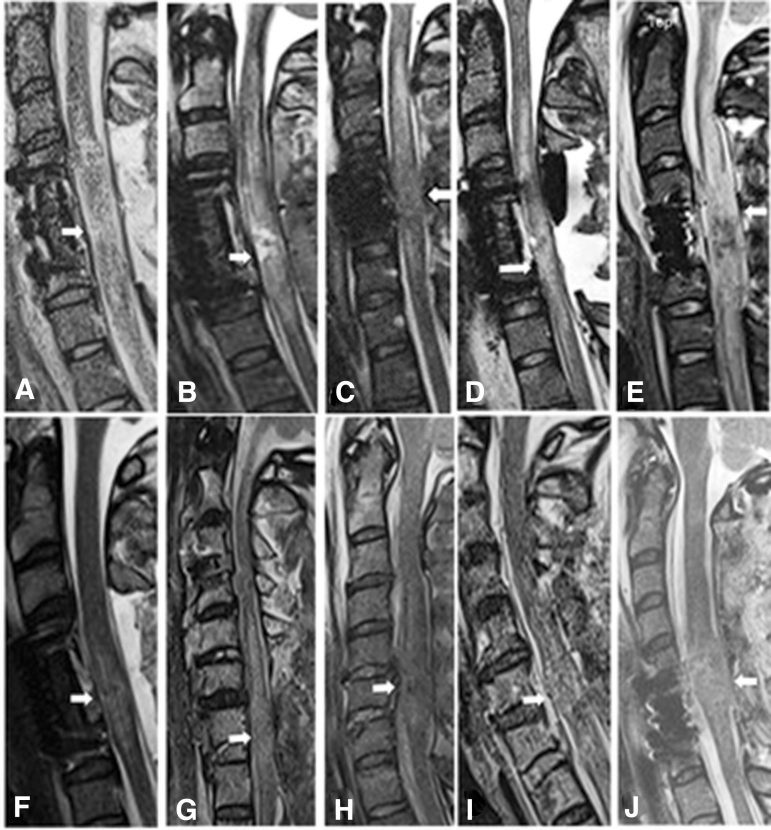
Post-operative midsagittal magnetic resonance imaging views from 10 patients with inadequate decompression despite anterior cervical corpectomy and fusion (ACCF) and laminectomy (plates A, B, D, E, F, J), anterior cervical discectomy and fusion, and laminectomy (plate C), and standalone laminectomy with posterior spinal fusion (plates G, H, I). The cerebrospinal fluid (CSF) is not visible either dorsal or ventral to the spinal cord indicating excessive swelling. Although intraspinal pressure was not unknown, in patients B and C, expansion duraplasty was performed to generate a CSF interface between the spinal cord and dura.
